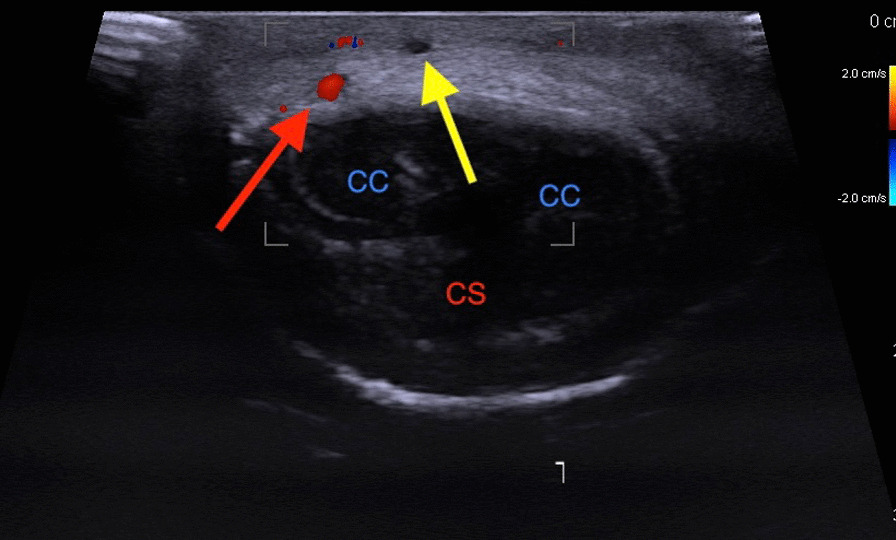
Doppler ultrasound of penis: cross section, CC—Corpus Cavernosum Penis. CS—Corpus Spongiosum Penis, Yellow Arrow—Dorsal Superficial penile vein without flow, Red Arrow—Right Dorsal penile artery
